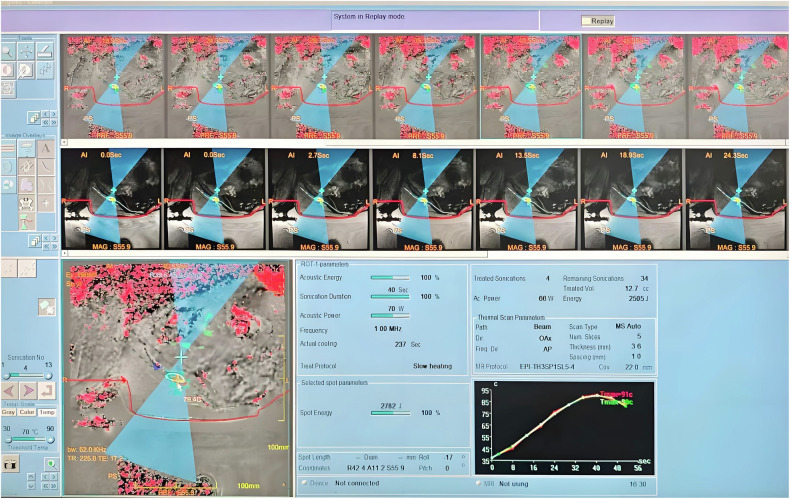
The actual working interface for temperature measurement during MRgFUS. This is a male patient with iliac bone metastasis, where the red line represents the skin surface, the white line indicates the bone cortex interface, the blue beam signifies the path of the ultrasound beam, the green circle denotes the actual treatment area, the yellow cursor marks the location for temperature measurement, and the curve in the bottom right corner represents real-time temperature changes in the monitored region
